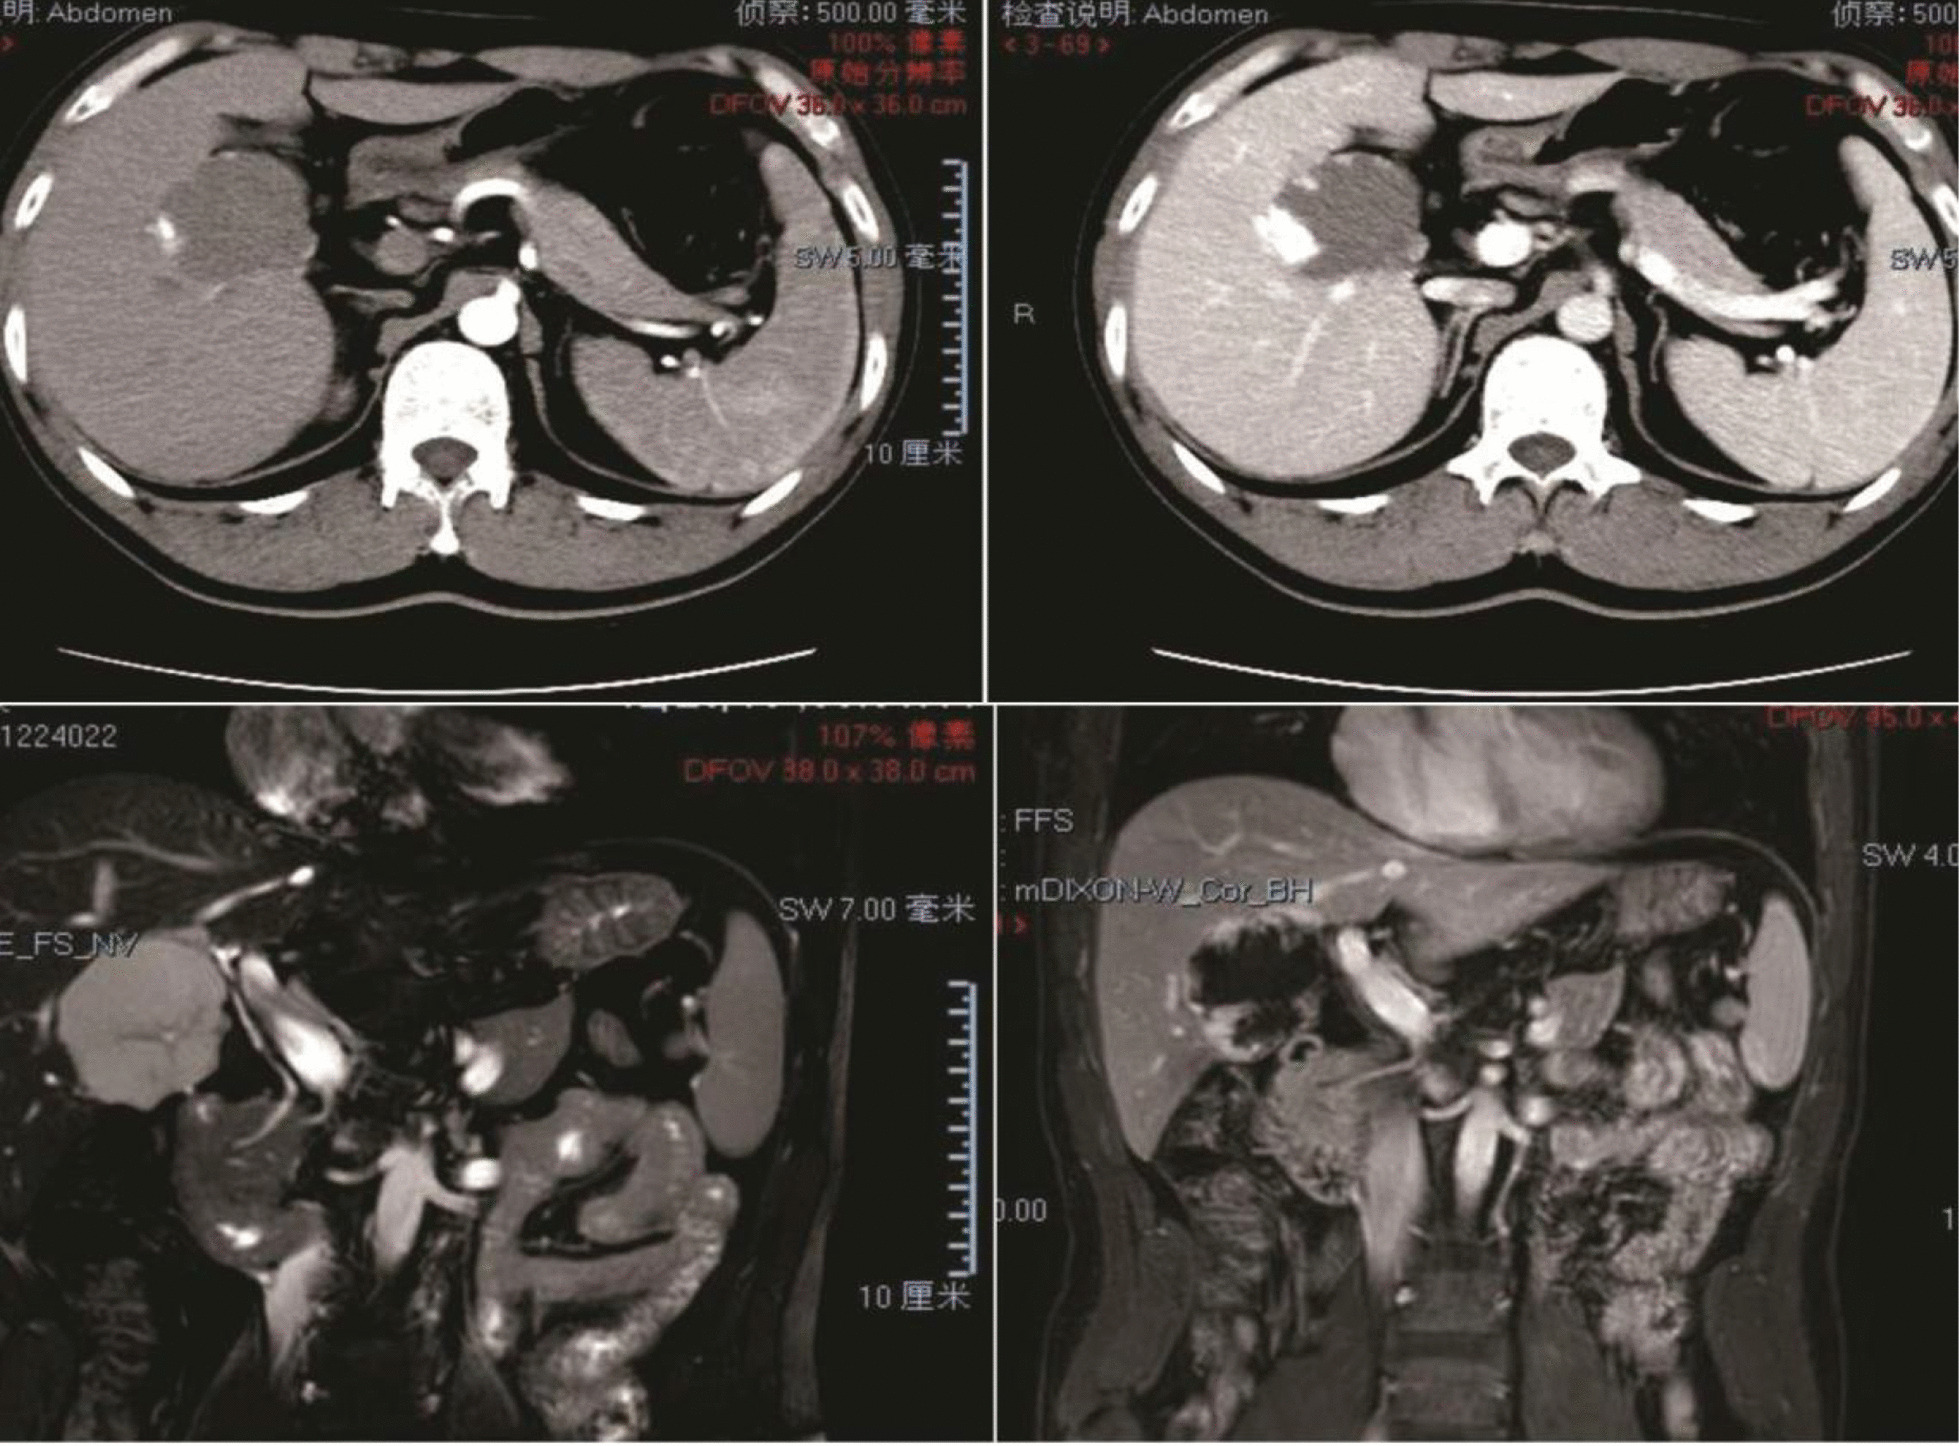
A 45-year-old male patient, a seaman, had symptoms of obstructive jaundice and was diagnosed with a hepatic hemangioma about 5 cm in diameter near the first porta hepatis. CT and MRI images show the hepatic hemangioma at the first porta hepatis, compressing the intrahepatic bile duct, which caused obstructive jaundice. We enucleated the tumor and the patient’s bilirubin level returned to normal
